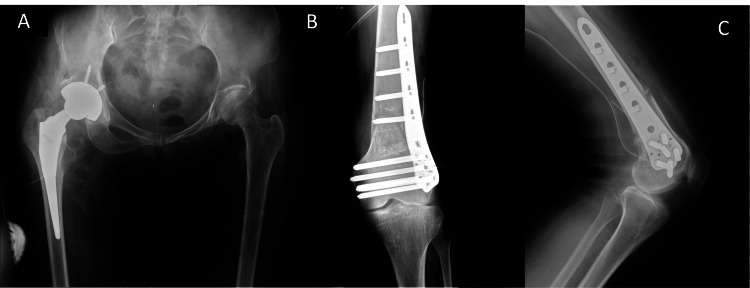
Postoperative radiographs Postoperative radiographs of the affected regions including the right hip and left knee joint. A) Anteroposterior radiograph at the hip. (B) Anteroposterior radiographs of the knee C) lateral radiograph of the knee.
